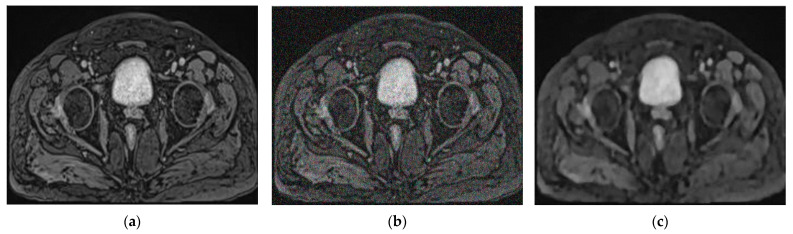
Examples of MRI images (a) original; (b) with Gaussian noise; (c) after applying residual learning.
